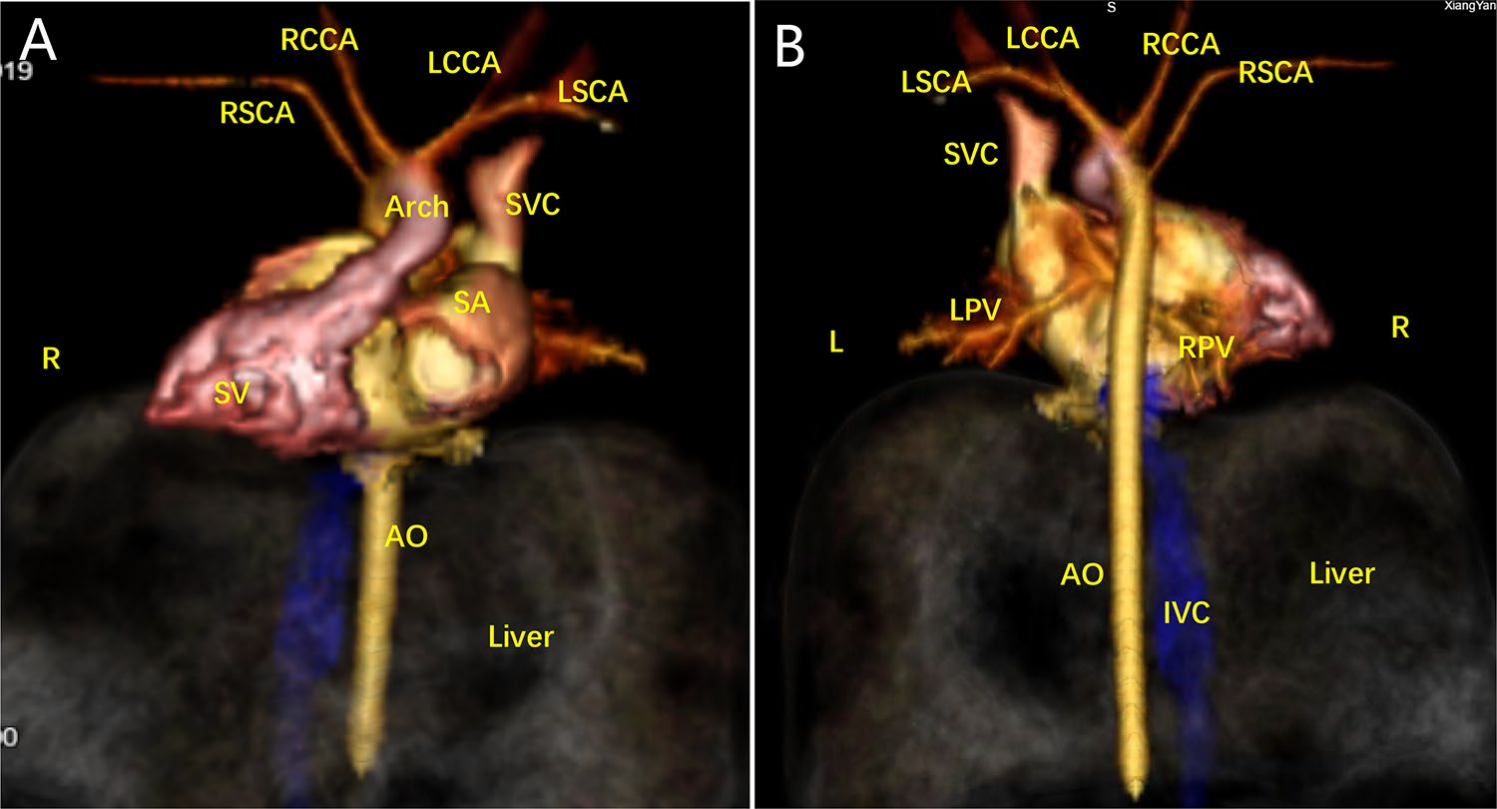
Postpartum CTA findings. (A–F) CTA revealed dextrocardia, a single ventricle, isolated left superior vena cava, right aortic arch with mirror branches, and left and right PVs returning to the left superior vena cava via vertical veins. *AO* ascending aorta, *IVC* inferior vena cava, *LCCA* left common carotid artery, *LSCA* left subclavian artery, *RCCA* right common carotid artery, *RSCA* right subclavian artery, *SA* single atrium, *SV* single ventricle.
